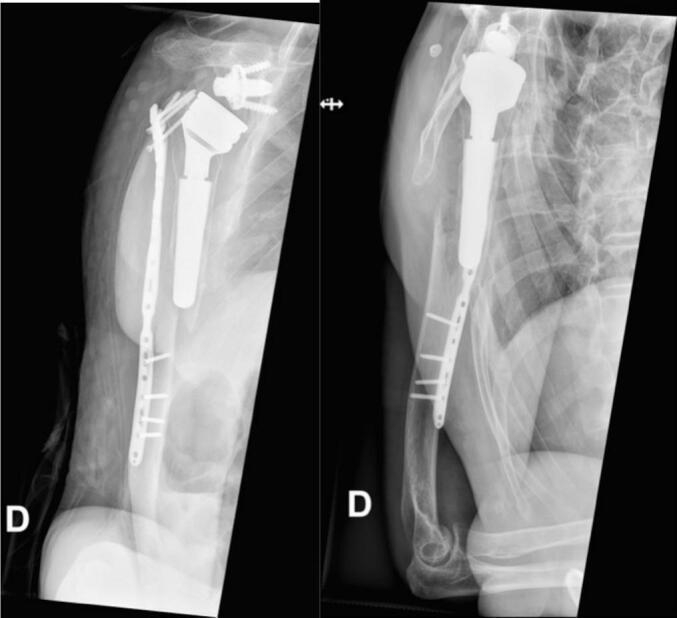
Postoperative radiographs showing the early loss of reduction after ORIF of the humeral periprosthetic fracture.
